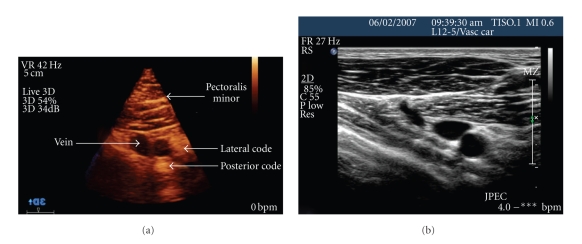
(a) Three-dimensional infraclavicular ultrasound image. (b) Two-dimensional infraclavicular ultrasound image.
